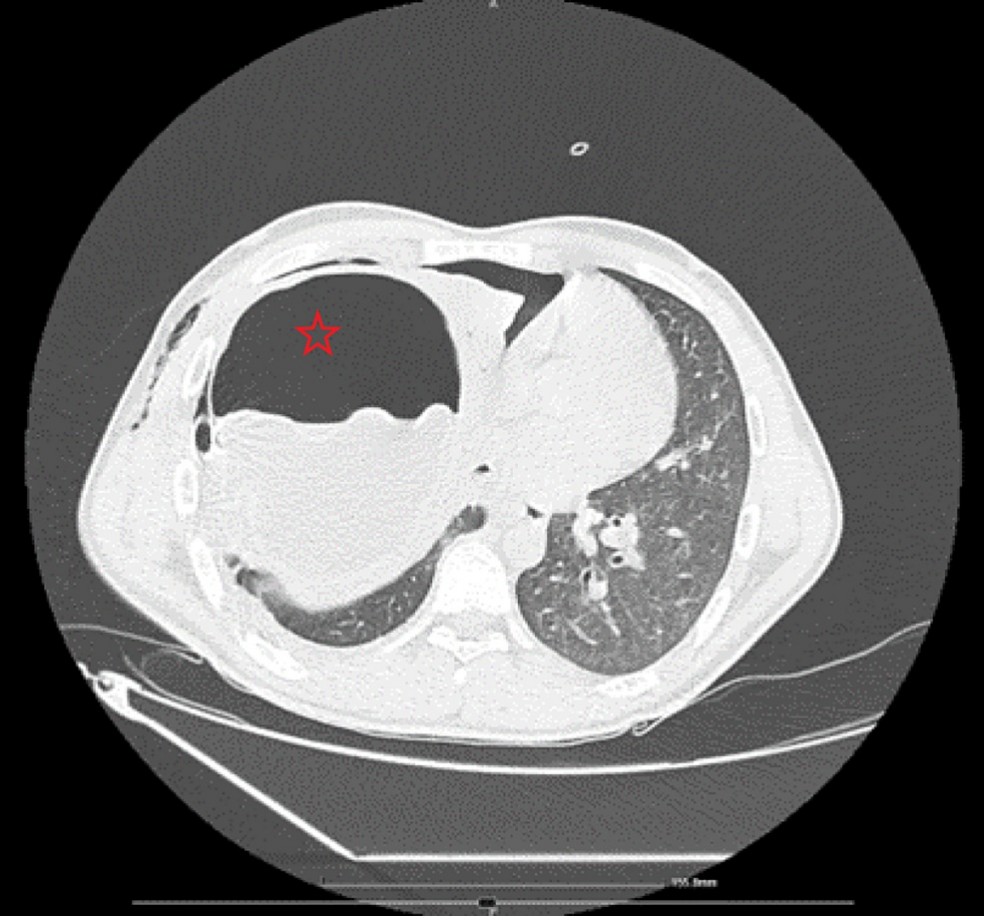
Chest CT of cystic lesion A large fluid-filled cyst and cyst rupture in the right lung.
